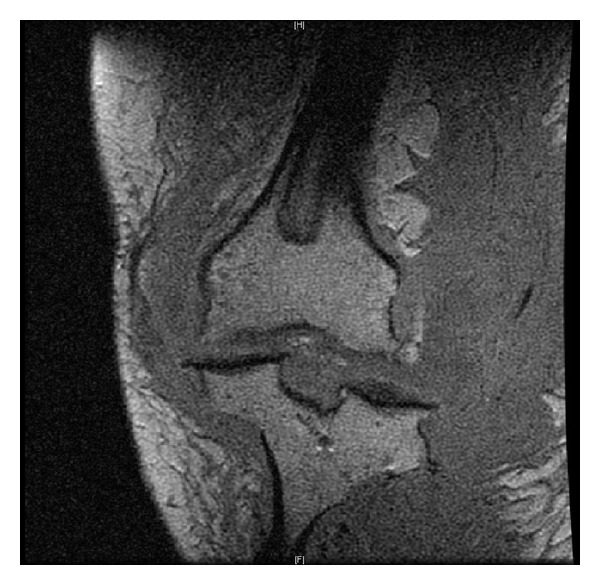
MRI demonstrates bony deformities of distal femur and proximal tibia.
